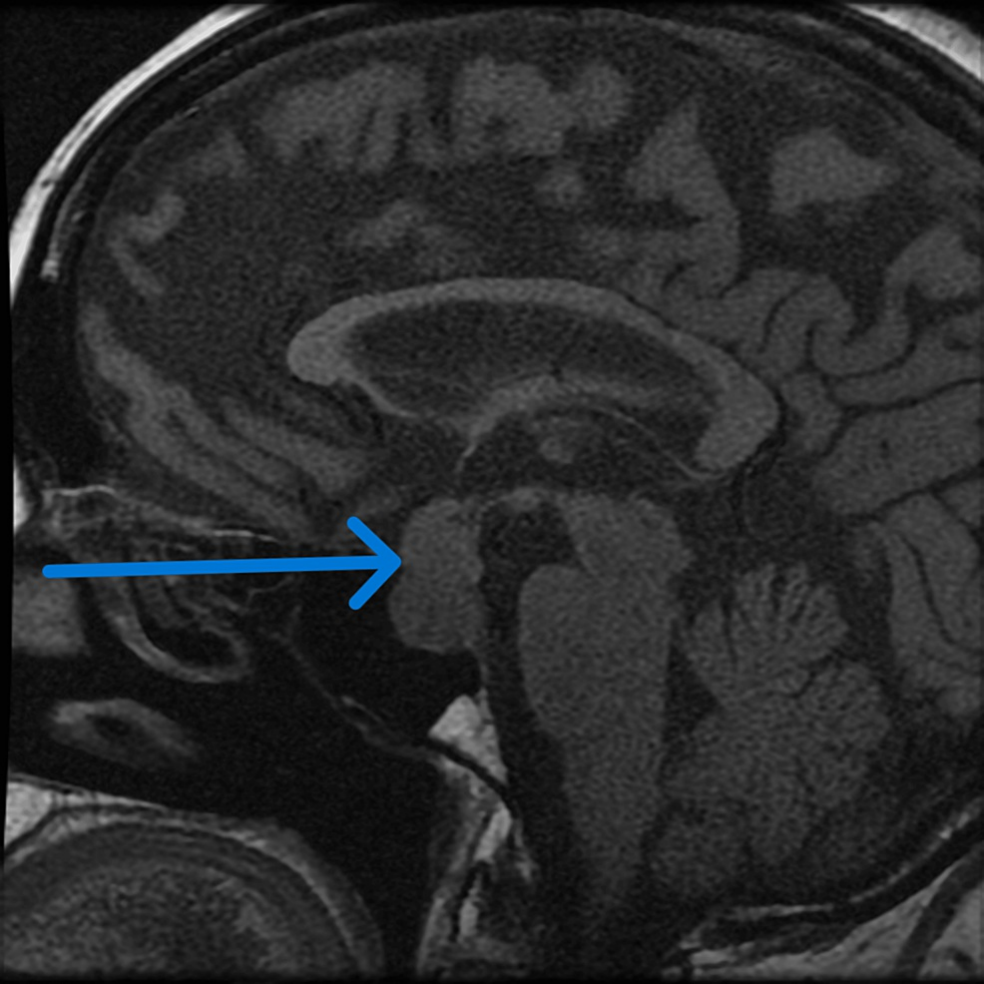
MRI of the pituitary gland in the coronal section The arrow shows the large pituitary tumor.
